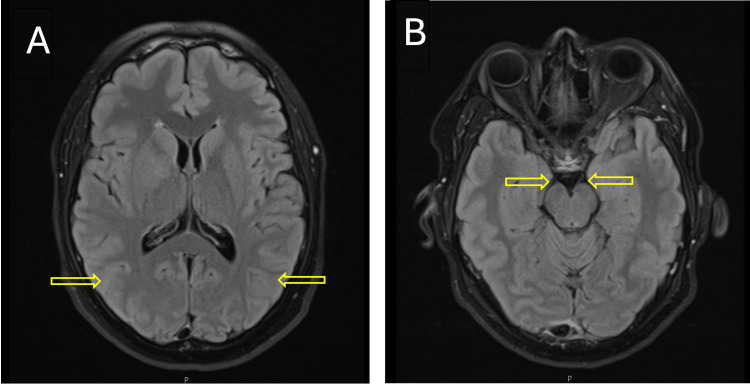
MRI of the head axial T2-weighted dark fluid MRI sequence. Axial T2-weighted dark fluid MRI sequence demonstrating no signal abnormalities in the occipital cortex or optic tracts. (A) No signal abnormalities in the occipital cortex bilaterally. (B) No signal abnormalities in the optic tracts bilaterally.
